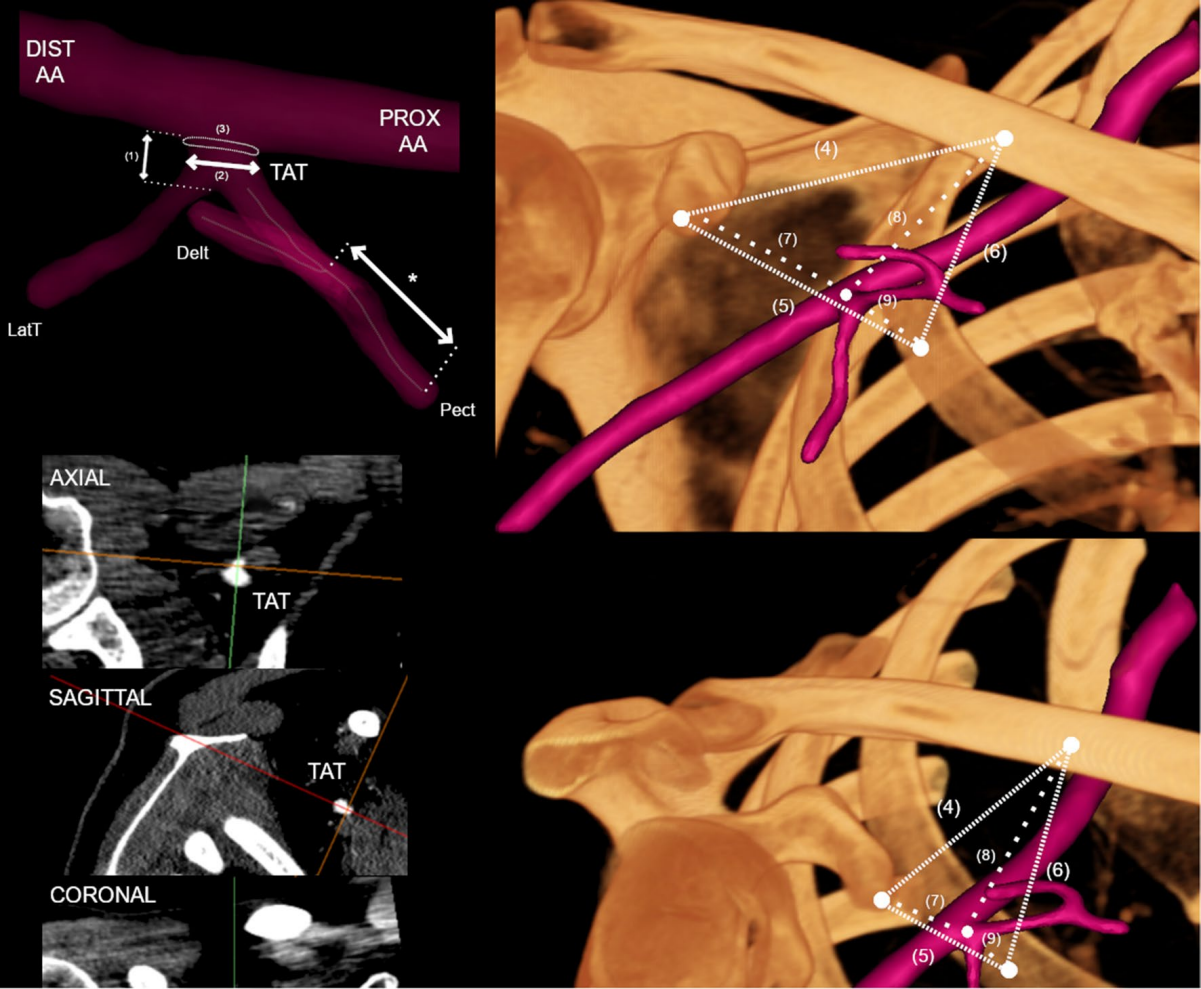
Measurement methodology presented in graphical form. To determine the TAT morphology, the multiplanar reconstruction of CT images was performed. *PROX AA* Proximal axillary artery; *DIST AA* distal axillary artery; *TAT* thoracoacromial trunk; *Delt* deltoid branch of TAT; *LatT* lateral thoracic artery; *Pect* pectoral branch of TAT; (1) Length of TAT; (2) maximal diameter of TAT; (3) TATs’ ostial area; (4) distance from the coracoid process to the clavicle, (5) distance from the coracoid process to the second rib, (6) distance from the clavicle process to the second rib, (7) distance from the coracoid process to the TAT, (8) distance from the clavicle to the TAT, and (9) distance from the second rib to the TAT. An asterisk (*) indicates the length of the corresponding consecutive Pect of TAT or its branches
